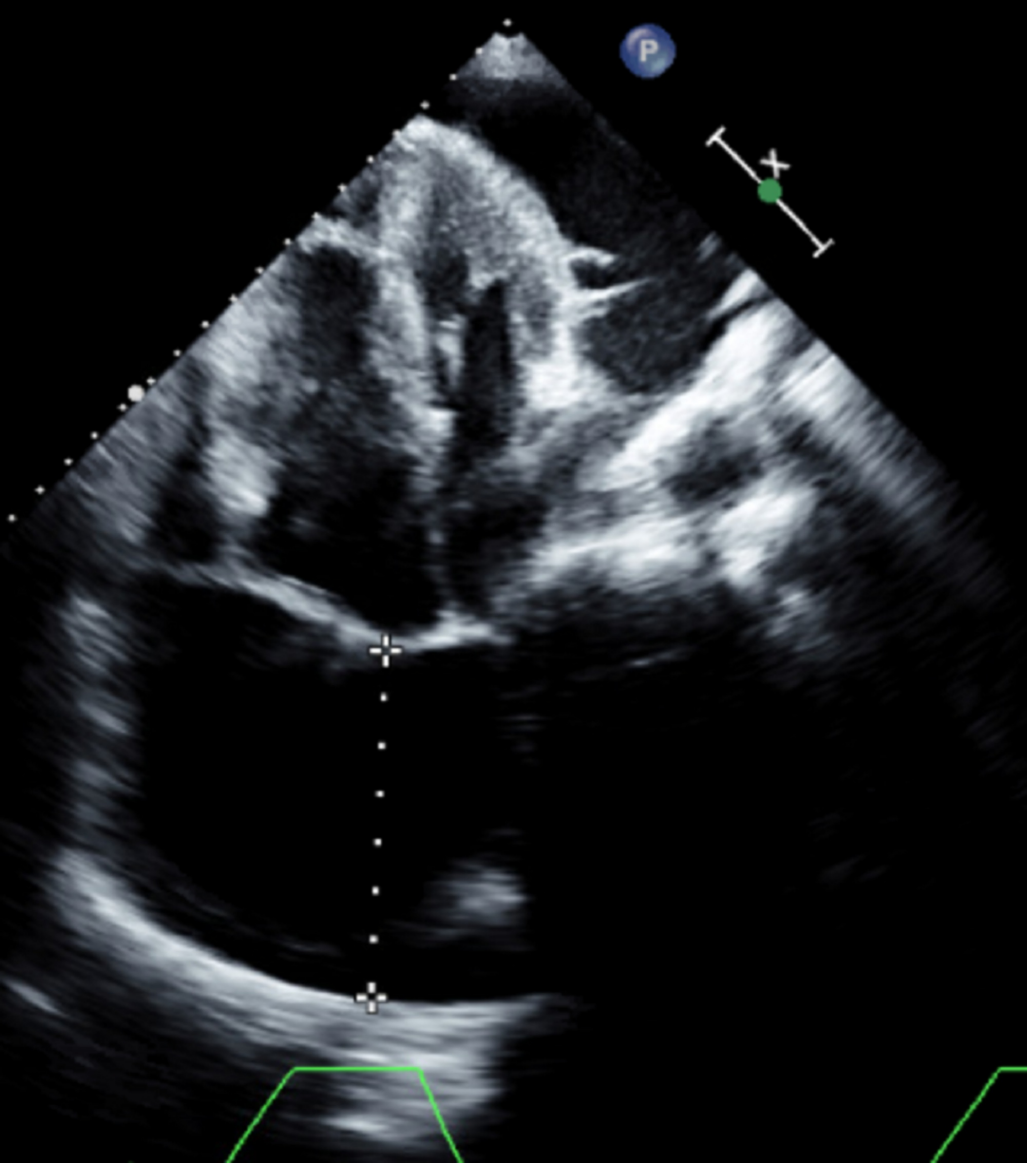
Apical four-chamber view by transthoracic echocardiogram at admission showing the large pericardial effusion
